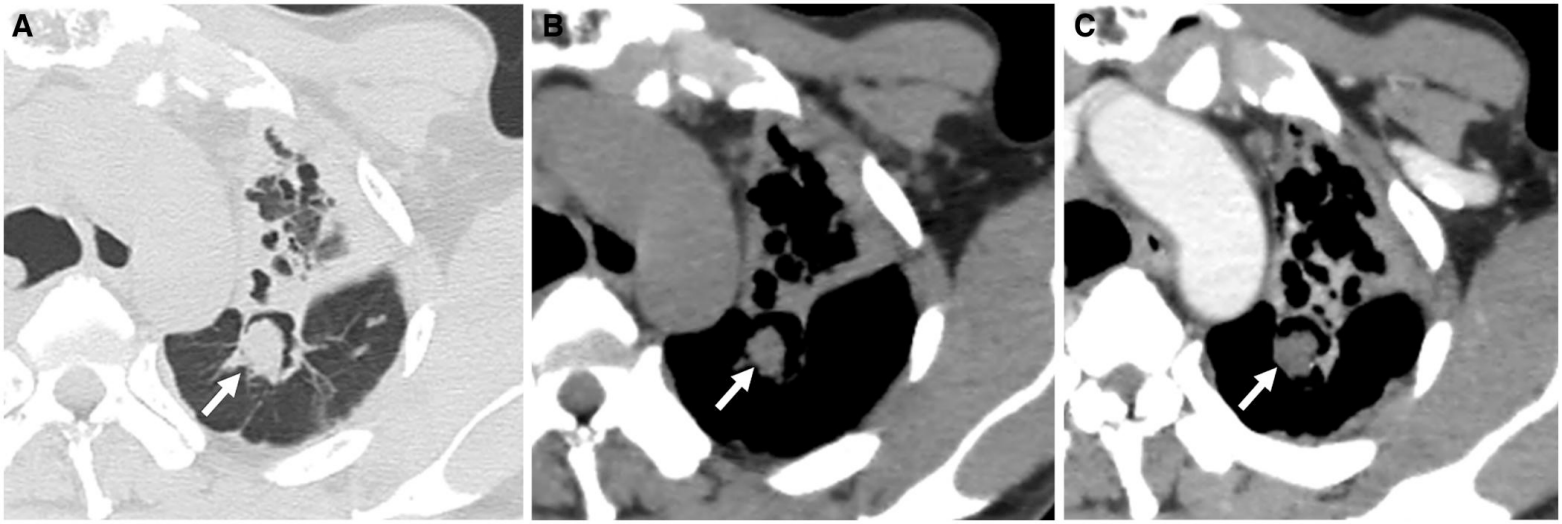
Aspergilloma within a tuberculous cavity in a 69-year-old man. (A–C) Axial CT images show a fungal ball (white arrows) within the upper lobe parenchymal cavity. (A) The lung window image shows a fungal ball in dependent position within a thick-walled cavity. There is an air crescent sign. Note extensive scarring around the cavity. (B) Unenhanced and (C) contrast-enhanced images show that the fungal ball does not enhance. This lack of enhancement helps distinguish a fungal ball from a tumour.
